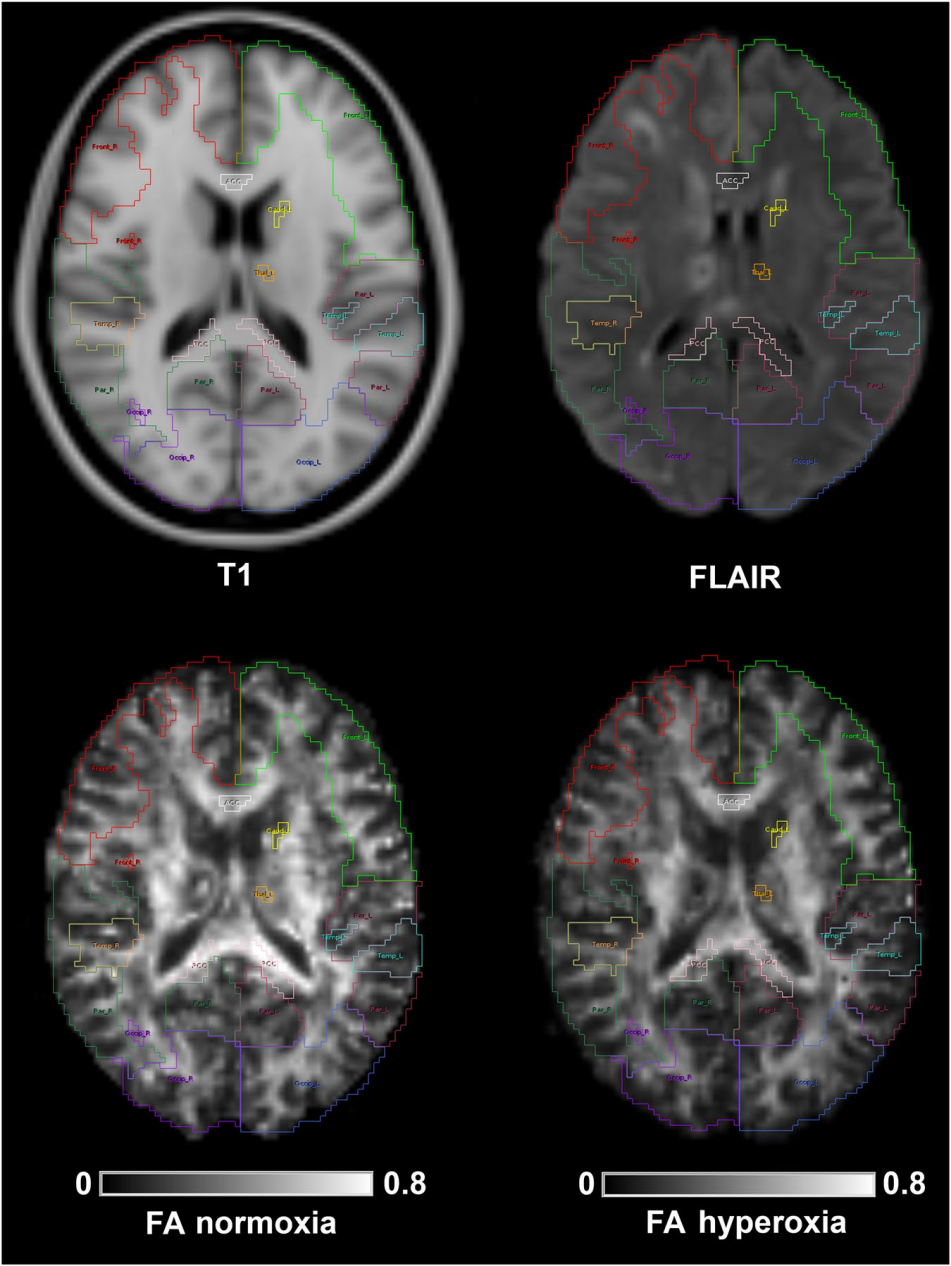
Individualised template regions of interest. Standard T1 weighted magnetic resonance image and patient fluid attenuated inversion recovery (FLAIR), with fractional anisotropy (FA) at baseline normoxia and following hyperoxia; all images are displayed in Montreal Neurological Institute 152 (MNI152) space. The region of interest (ROI) template for this subject (subject 1) has been individualised by the exclusion of lesion core and contusion tissue. On the FLAIR image slice shown lesions can be seen within the right frontal and temporal cortex, white matter, right caudate and right thalamus. On this axial slice regions shown include frontal left (Front_L), frontal right (Front_R), temporal left (Temp_L), temporal right (Temp_R), parietal left (Par_L), parietal right (Par_R), occipital left (Occip_L), occipital right (Occip_R), anterior corpus callosum (ACC), posterior corpus callosum (PCC), caudate left (Caud_L) and thalamus left (Thal_L).
